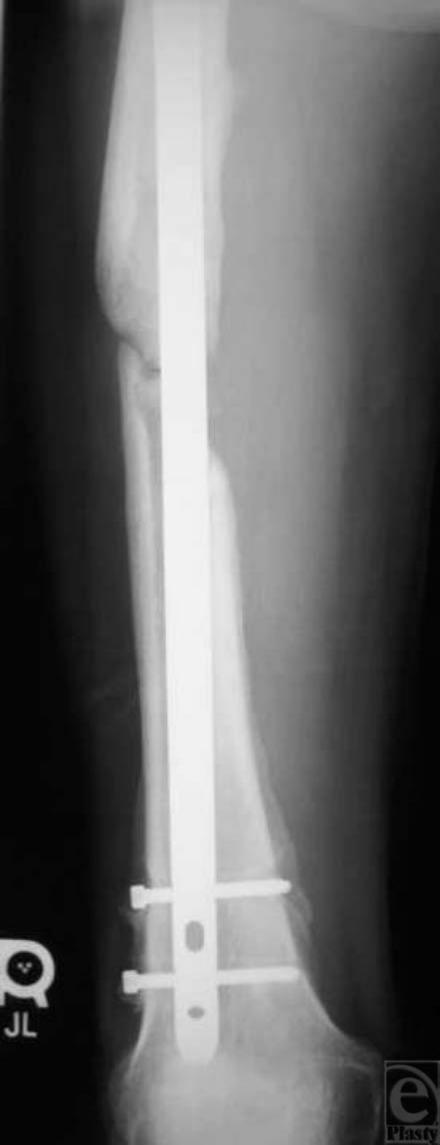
Anteroposterior radiograph approximately 1 year after injury shows atrophic nonunion of the fracture.
